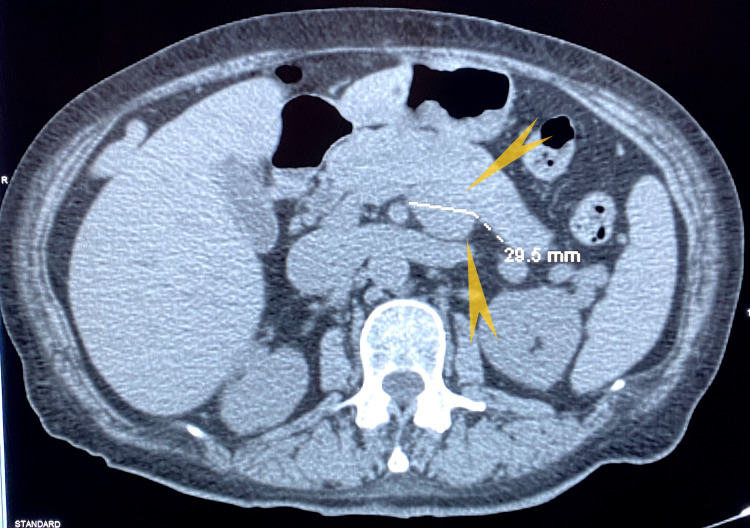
CT abdomen and pelvis w/contrast: There is a diffuse mass-like enlargement of the mid-body of the pancreas with 3 cm lobular mass projected from the superior and inferior aspects of the pancreas. Findings are highly suspicious for pancreatic neoplasm.
